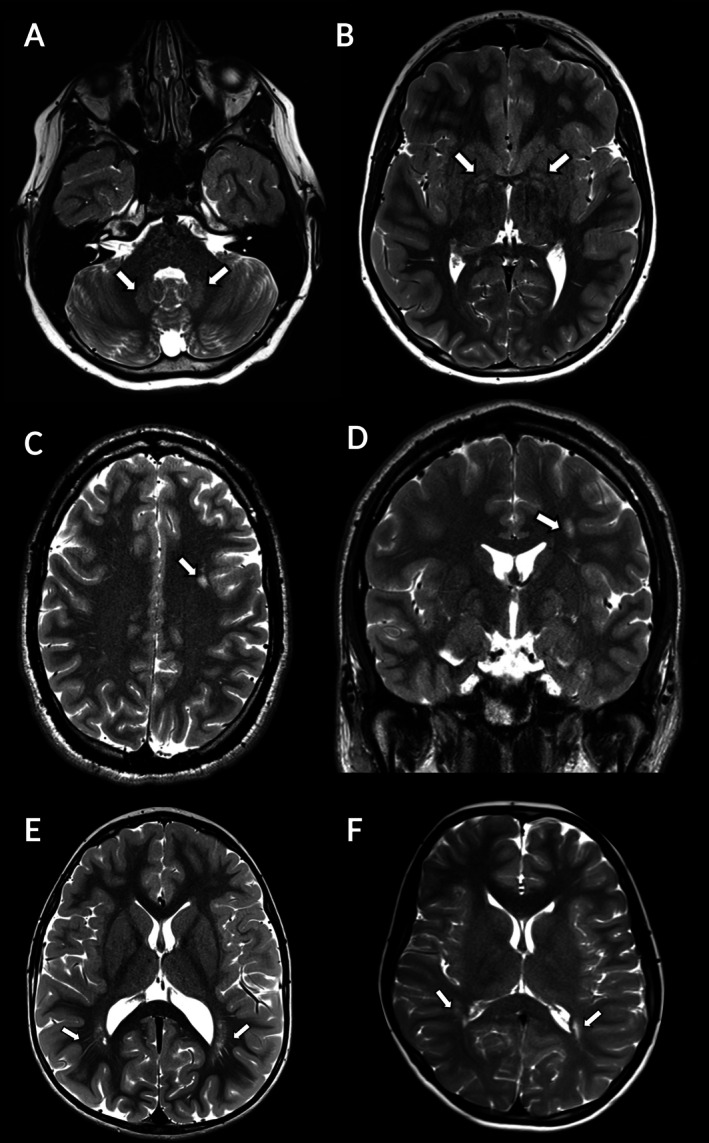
Myelination abnormalities in individuals with succinic semialdehyde dehydrogenase deficiency (SSADHD), as demonstrated by signal hyperintensities (white arrows) in T2‐weighted MRI images in the dentate nuclei (A) and globus pallidi (B) of a 9‐year old individual, subcortical white matter of the left frontal lobe (C, D) of a 24 year‐old individual, and posterior peri‐atrial regions (E, F) of a 3‐year old individual.
